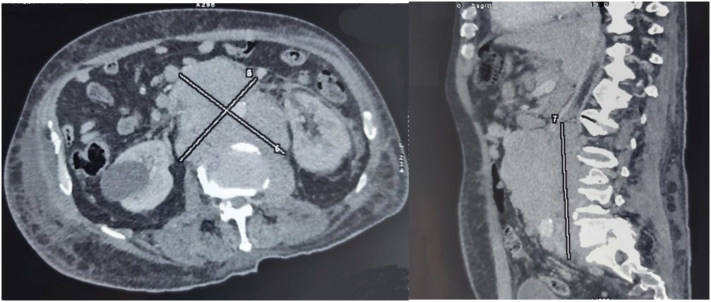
Voluminous retroperitoneal lymph nodes in transversal and sagittal views of thoraco-abdomino-pelvic computed tomography, realised 4 weeks after orchidetomy.
